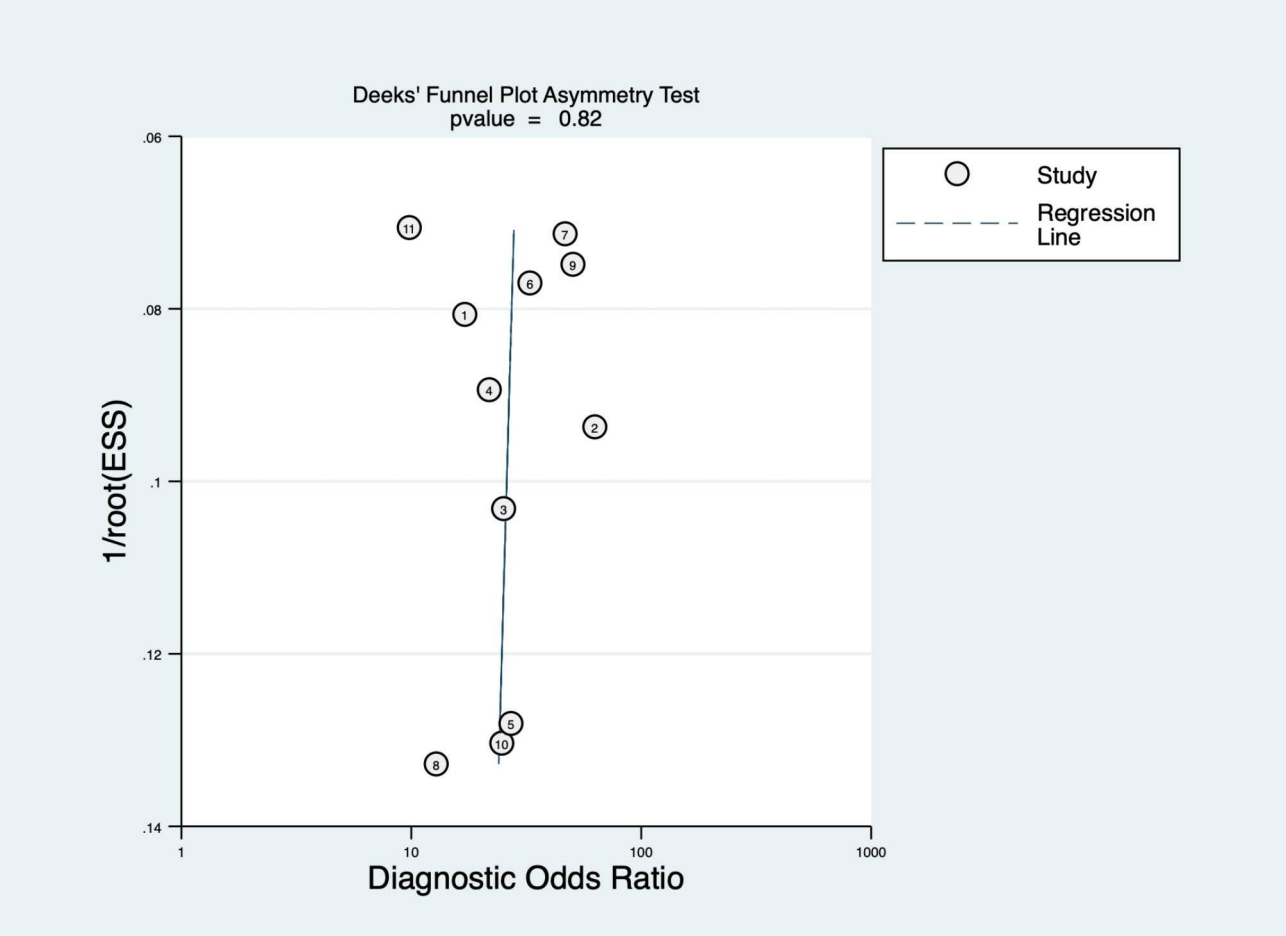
Funnel plot of MRI based on artificial intelligence algorithm for predicting lymph node metastasis in breast cancer patients
